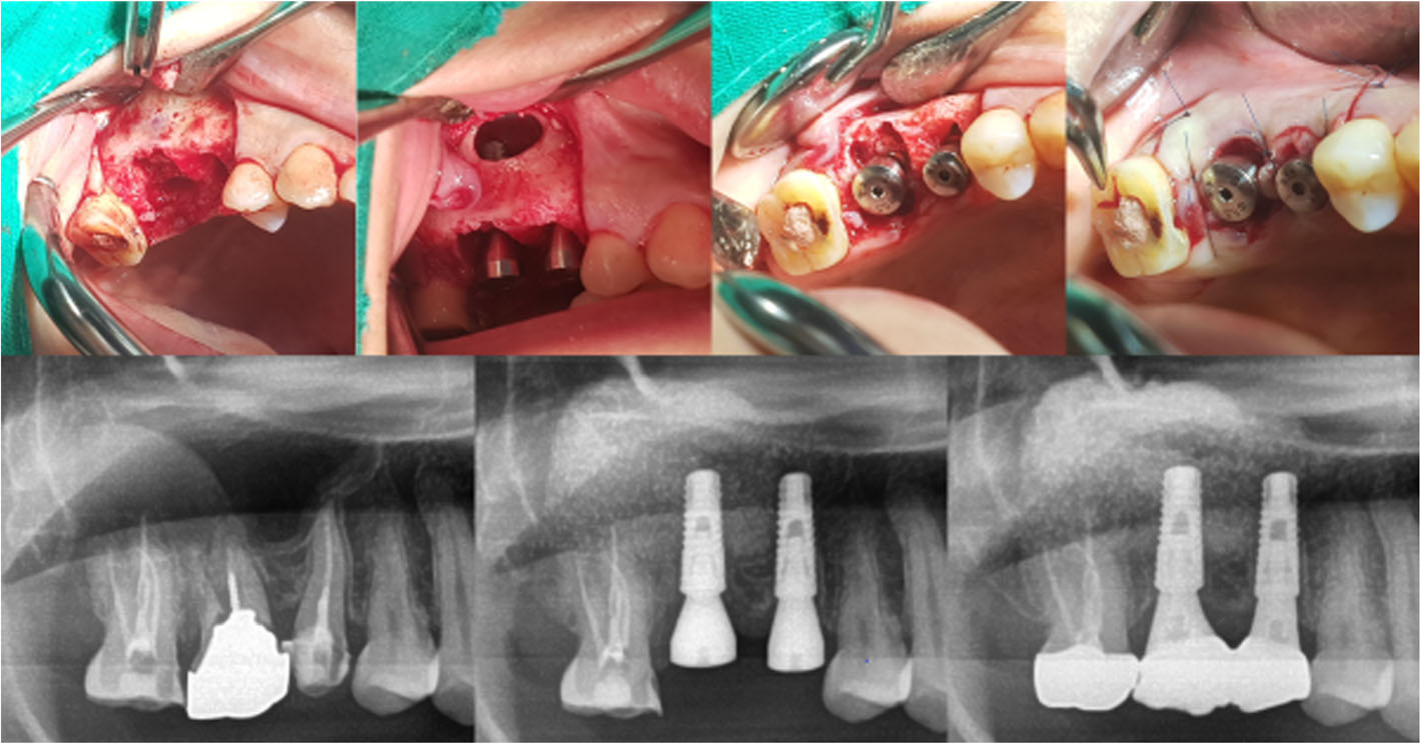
Clinical and radiographic aspects of immediate implant placement following tooth extraction with simultaneous lateral sinus lift: 1-stage (non-submerged)
